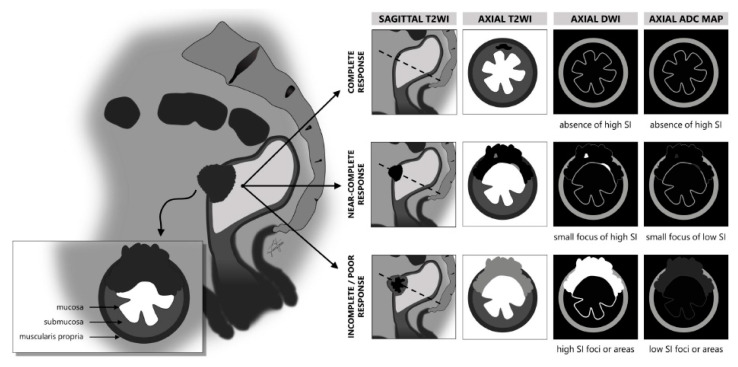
MRI-based morphologic response categories in rectal cancer. This figure delineates the newly introduced terminology by the Society of Abdominal Radiology Colorectal and Anal Cancer Disease-Focused Panel for classifying morphologic responses in colorectal and anal cancers using MRI. The visual guide showcases the following: Complete Response: characterized by the remarkable vanishing of intermediate signal intensity on T2-weighted imaging (T2WI), suggestive of substantial tumor size reduction, often seen as a scar in the mucosal or submucosal layers or normalized rectal wall structure; Near-Complete Response: indicative of significant tumor regression, near-complete response is a state that may evolve into a complete response with time. This stage may exhibit minimal but identifiable diffusion restriction; Incomplete Response: marked by a decrease in tumor volume with a residual detectable tumor on imaging, signifying persistent diffusion restriction and intermediate T2 signal within the tumor bed, necessitating further treatment consideration. Each category is supported by corresponding MRI findings (T2WI, diffusion-weighted imaging (DWI), and DWI-derived apparent diffusion coefficient (ADC) map findings) to aid in the comprehensive evaluation and accurate classification of treatment response.
